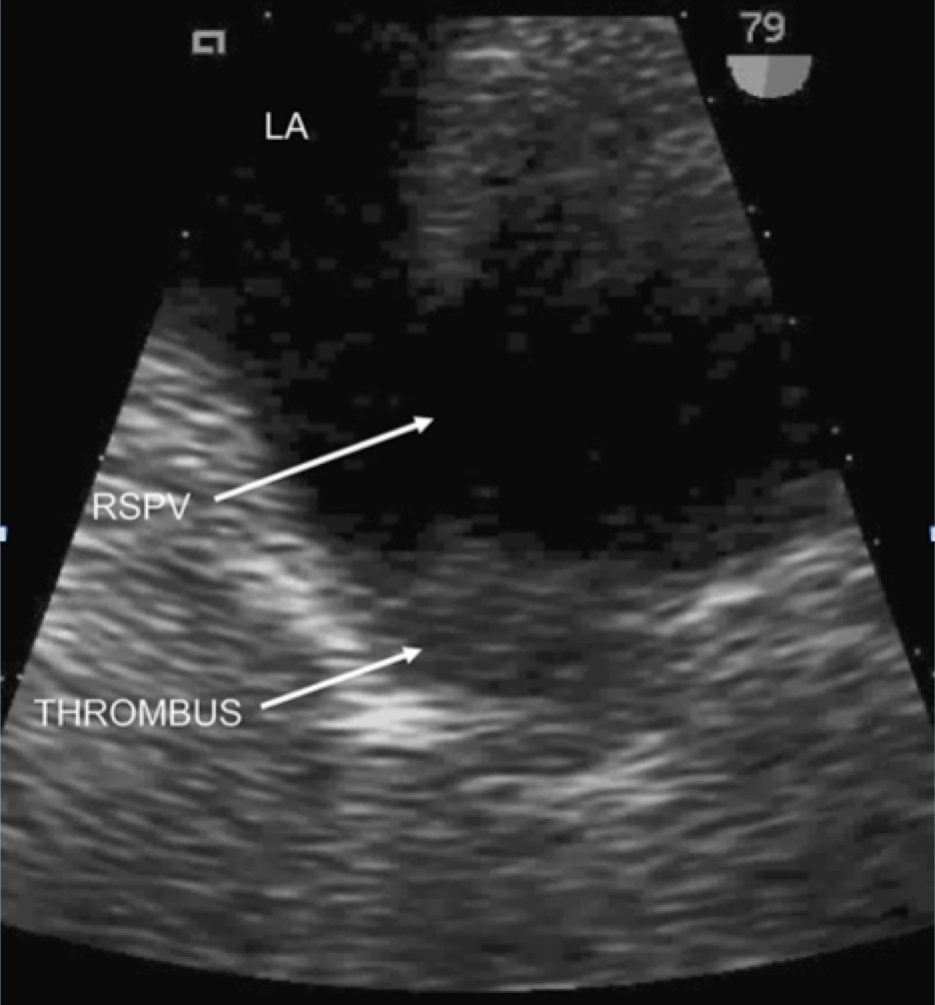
Baseline 2D transesophageal echocardiography TEE demonstrating mobile echogenic thrombus within right superior pulmonary vein. LA = left atrium; RSPV = right superior pulmonary vein; THROMBUS = pulmonary vein thrombus [36].
